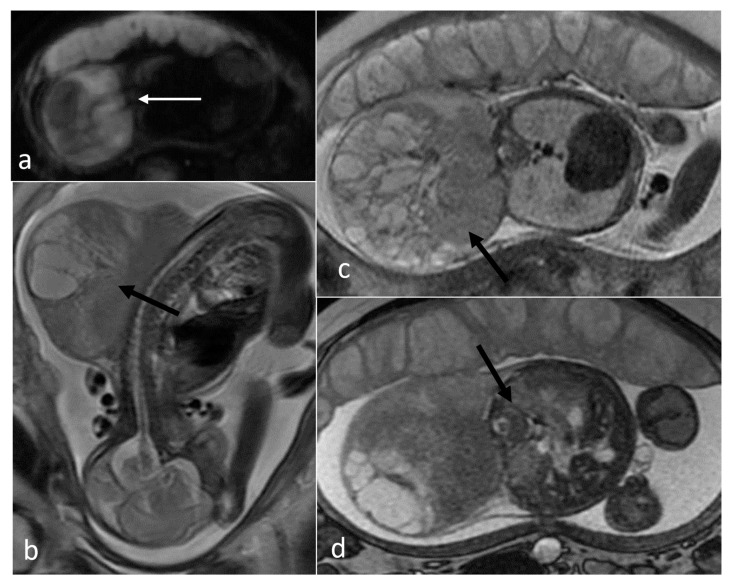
Infantile fibrosarcoma (arrows) in a fetus at a GA of 29 weeks. Huge heterogenous solid and cystic back mass infiltrating vertebral arches. (a) Axial DWI; (b) sagittal SSFSE/T2WI; (c) axial SSFSE/T2WI; (d) axial FIESTA.
